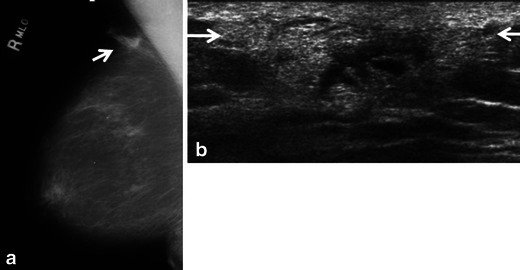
A 72-year-old woman with marginal zone B-cell lymphoma with follicular colonisation presents with developing focal asymmetry within the right upper breast noted on screening mammogram. a. Ill-defined focal asymmetry noted on the right medio-lateral oblique screening mammogram (*arrow*). b Ultrasound shows an irregular principally hyperechoic mass (*arrows*) extending to involve the skin with central areas of hypoechogenicity
